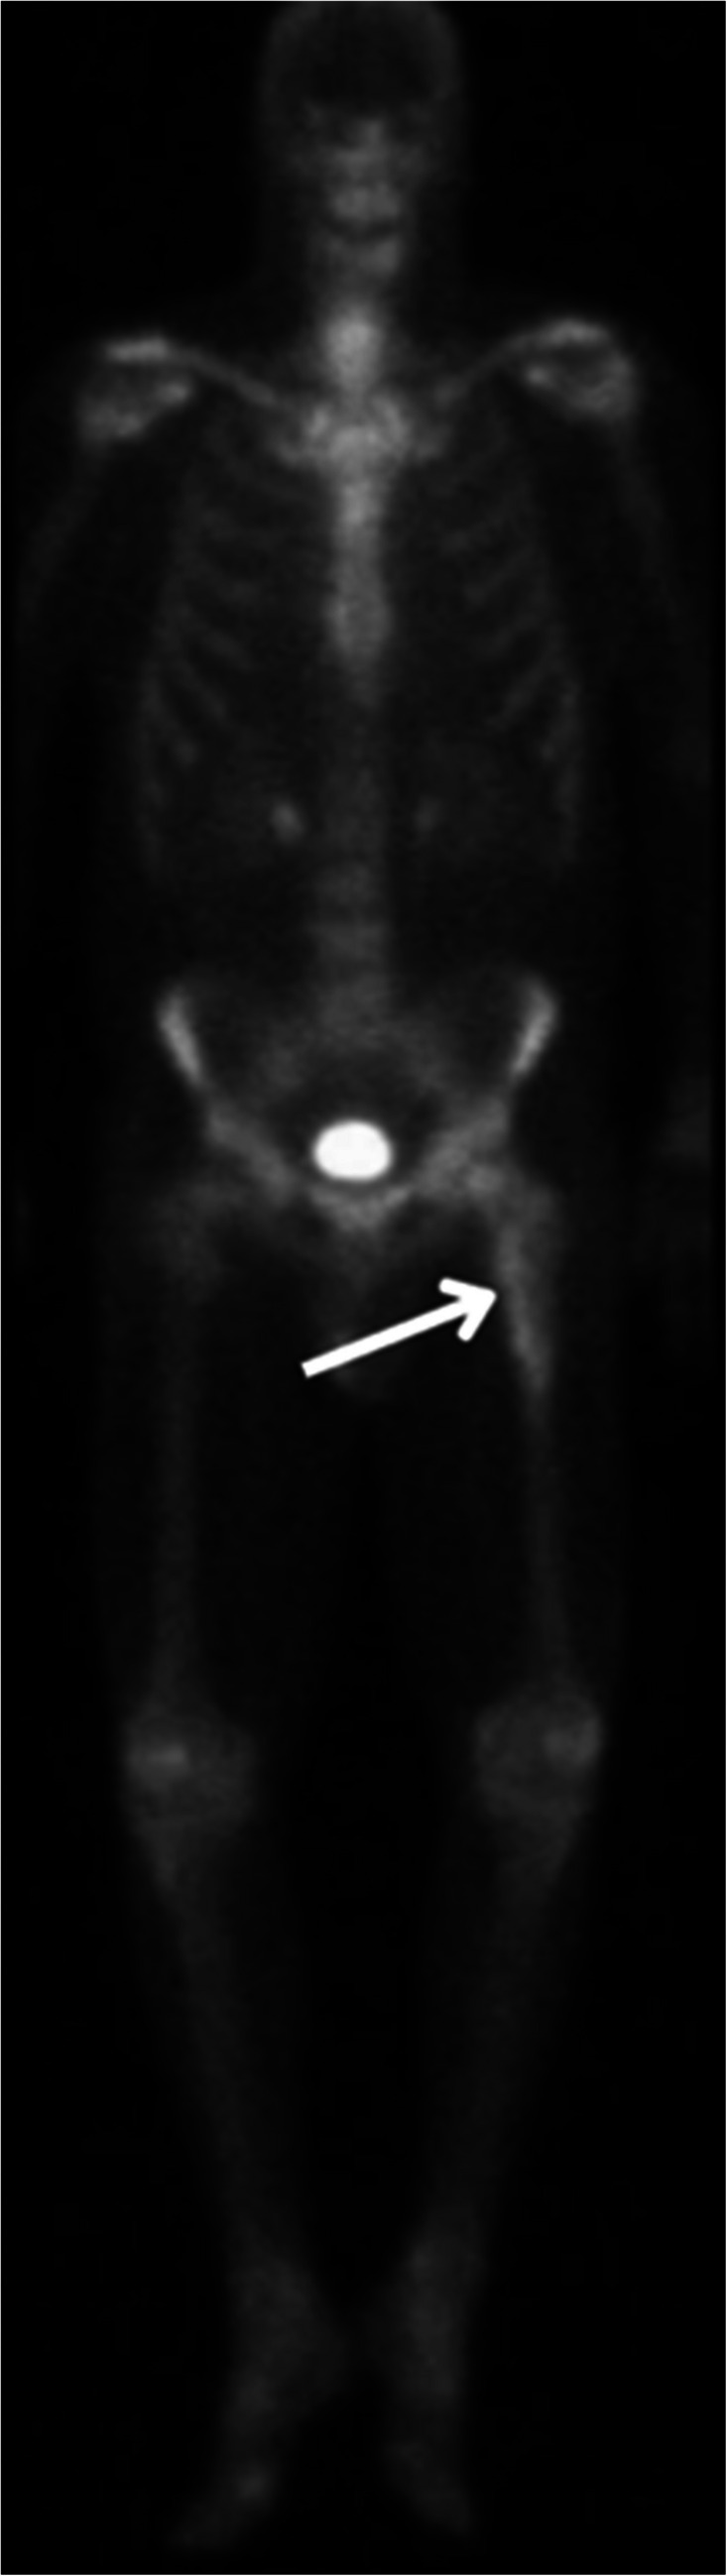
Anterior-posterior whole body view from bone scintigraphy reveals an elongated area of increased uptake of radionuclide extending from subchondral proximal femur to the proximal diaphysis with a flame shape inferiorly
